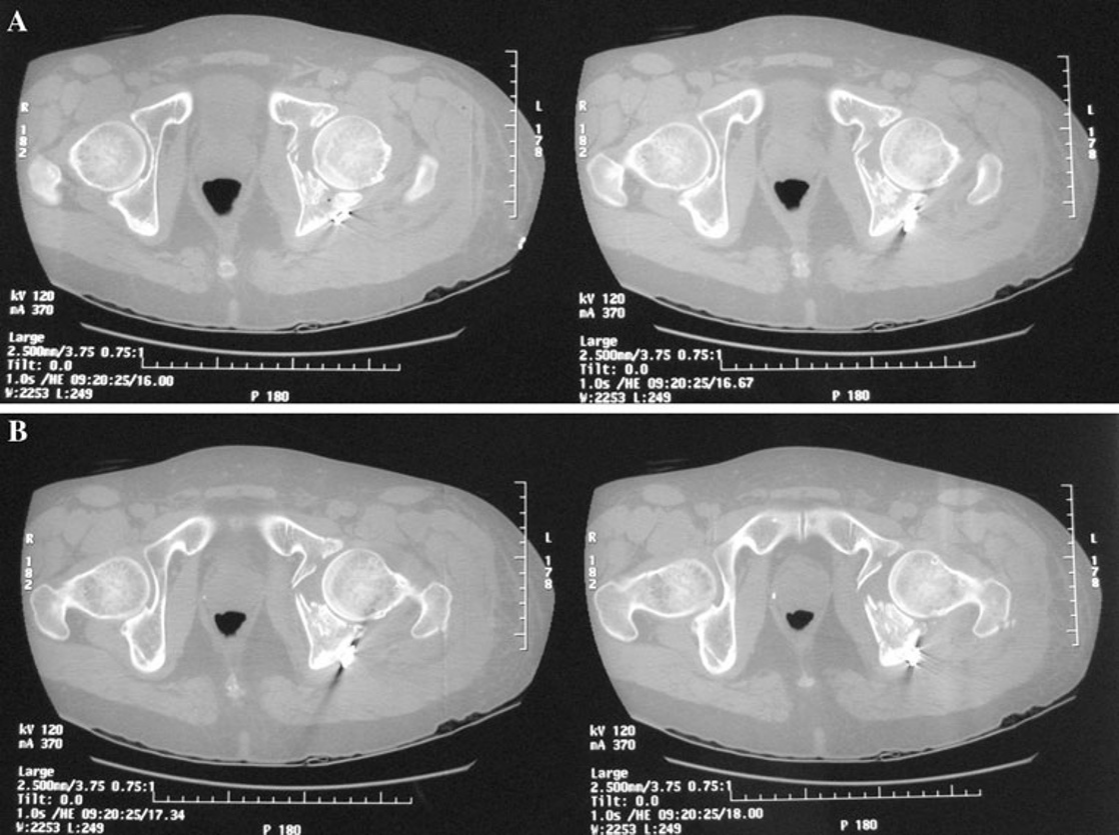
Postoperative computed tomography highlighting reduction of the intra-articular impacted fragment
